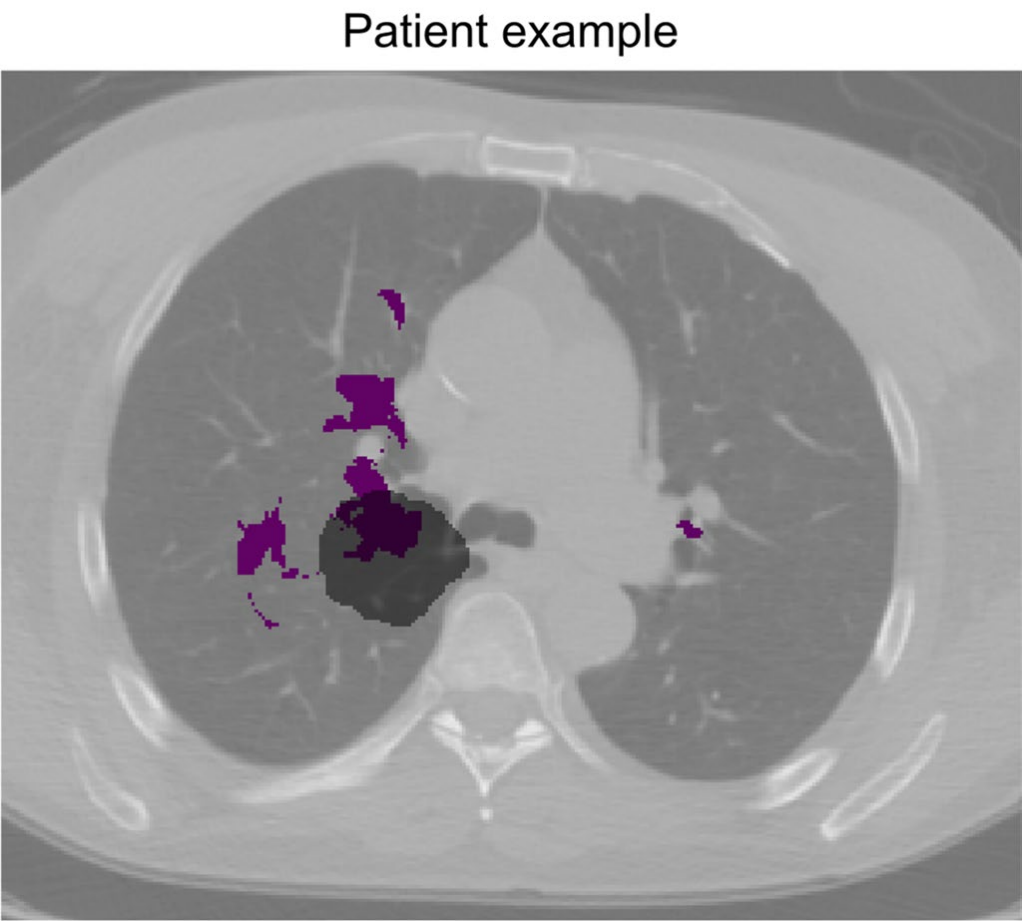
Example of an RCT2 patient with the primary tumor shown in gray and RCT1-decreased survival areas (DSA) shown in violet on an axial CT slice of the reference patient.
